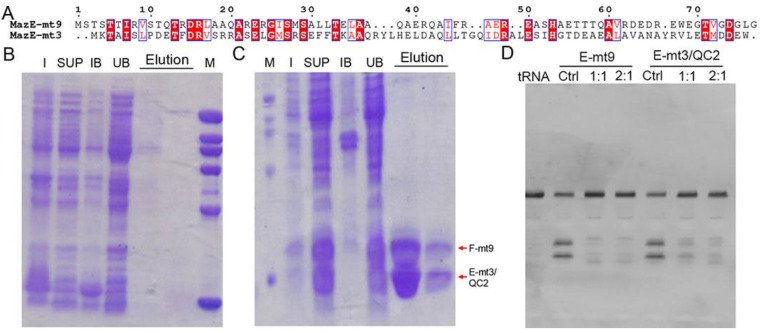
The investigation of the possible heterologous interactions between MazE-mt3 and MazF-mt9 and the engineering of MazE-mt3. (A) The sequence alignment between the MazE-mt9 sequence and that of MazE-mt3. (B,C) The first (B,C) second round of engineering of the MazE-mt3, and its subsequent co-expression profiles with MazF-mt9, which were named MazE-mt3/QC1 and MazE-mt3/QC2, respectively. M: molecular marker; I: Whole cell lysate of induced cells; SUP: the supernatant of ultrasonication after centrifugation; IB: the inclusion body after ultrasonication and centrifugation; UB: the unbound part from Ni-NTA column; Elution: the eluted fractions from the Ni-NTA column. (D) The inhibition against the tRNA-cleavage activity of the MazF-mt9 toxin by the engineered MazE-mt3/QC2 protein. Ctrl: MazF-mt9 without corresponding peptides. The ratios represented the molar ratios of toxin to various peptides used in the experiments.
